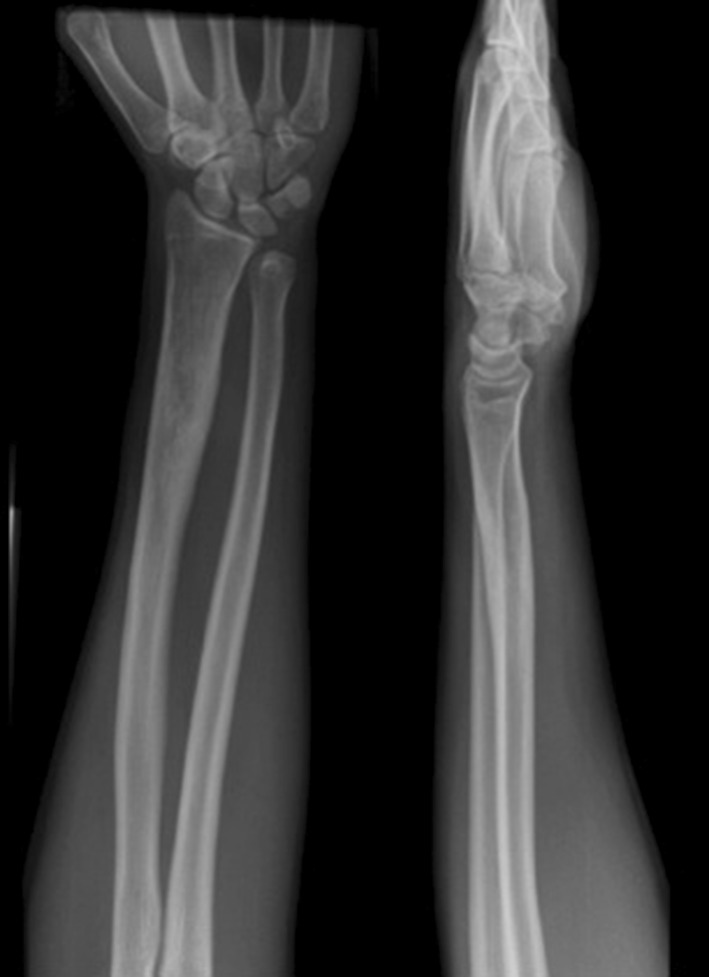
Radiographs 1 year after curettage of Brodie’s abscess
